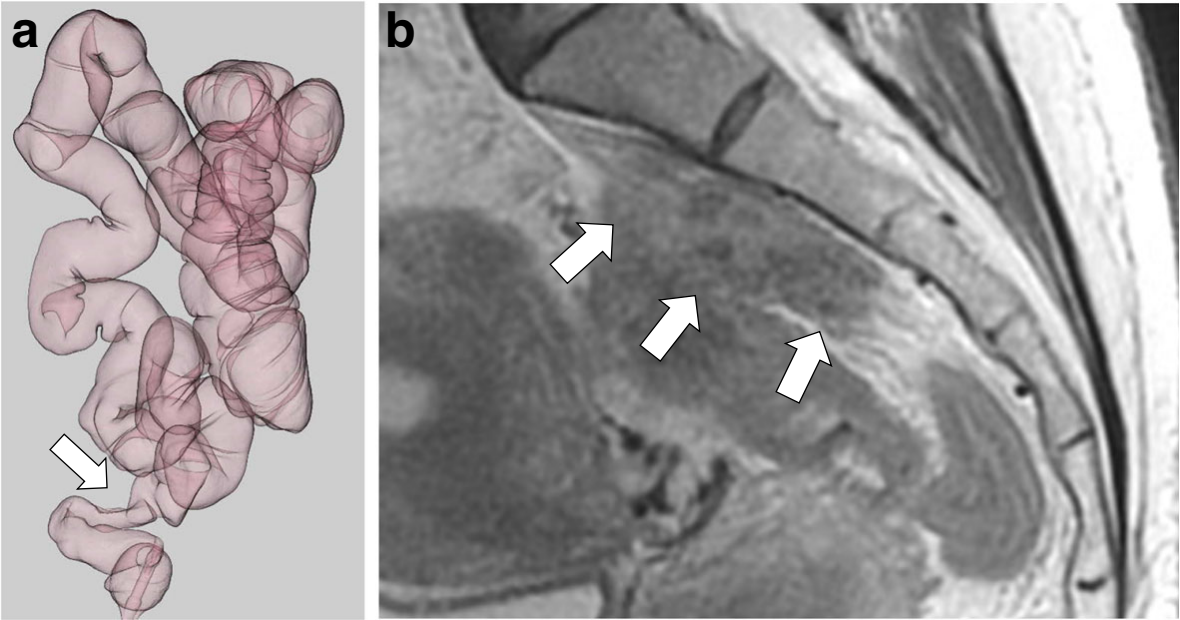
a CT colonoscopy revealed extrinsic compression of rectum. b Coronal magnetic resonance imaging (MRI) showed 4-cm low-intensity tumor between rectum and sacrum
